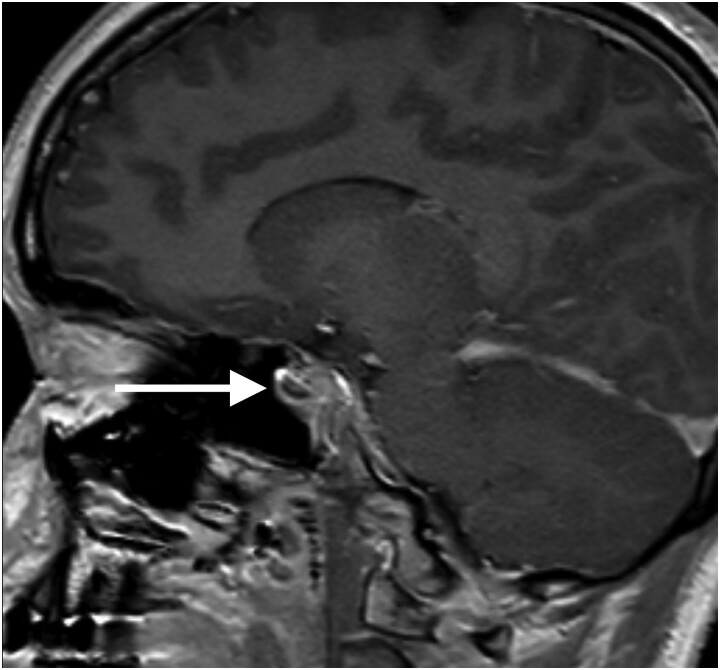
Magnetic resonance imaging of the pituitary showing mild enlargement with loss of normal posterior signal.
